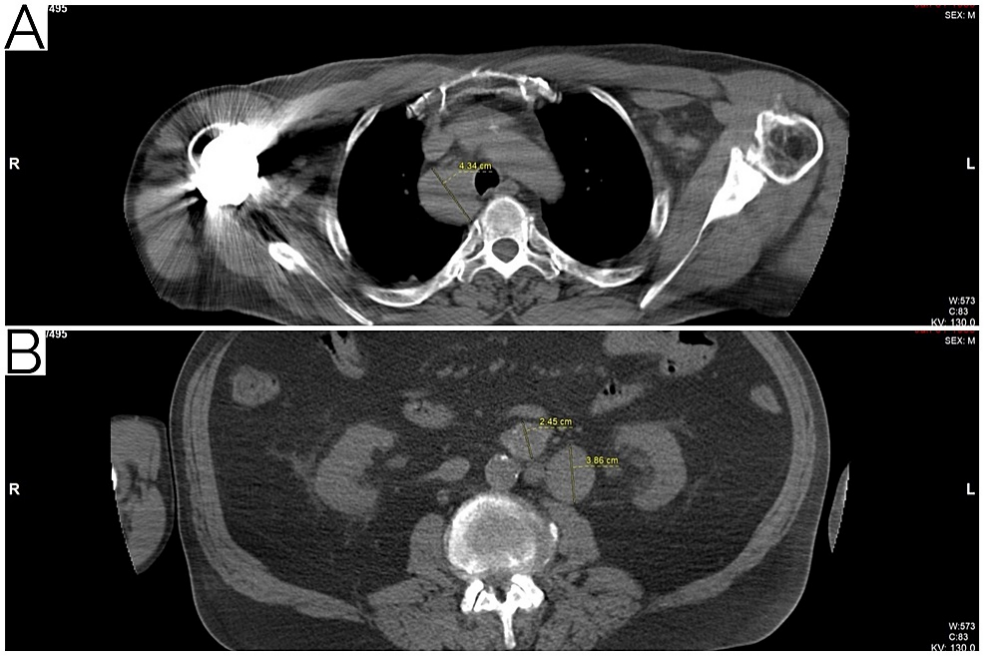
Baseline radiology. Baseline radiology of pathologic lesions, (A) right paratracheal mass and (B) left retroperitoneal mass, on CT correlates of PET imaging. CT, computed tomography; PET, positron emission tomography.
